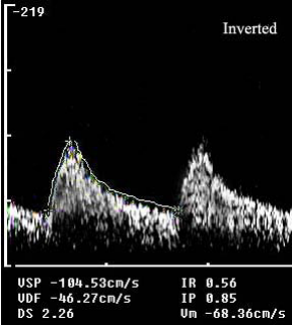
Two hours later, the transcranial Doppler showed a stenotic flow at the level of the basilar artery: a focal mean flow velocity increase >30% compared with the proximal arterial segment.
